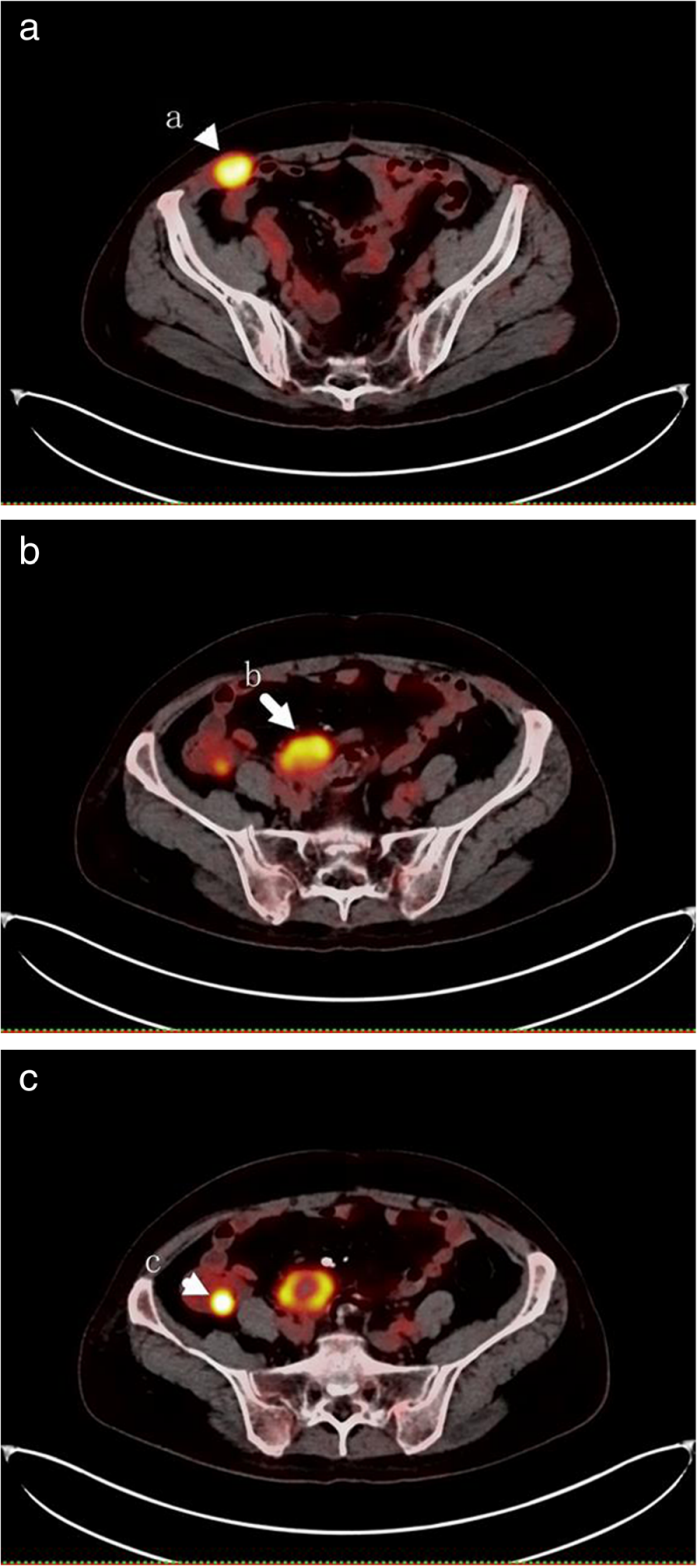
PET-CT fingdings. The elevated uptake of FDG in the right abdominal wall (a), multiple soft tissue masses around both iliac vessels (b) and ileocecus (c)
